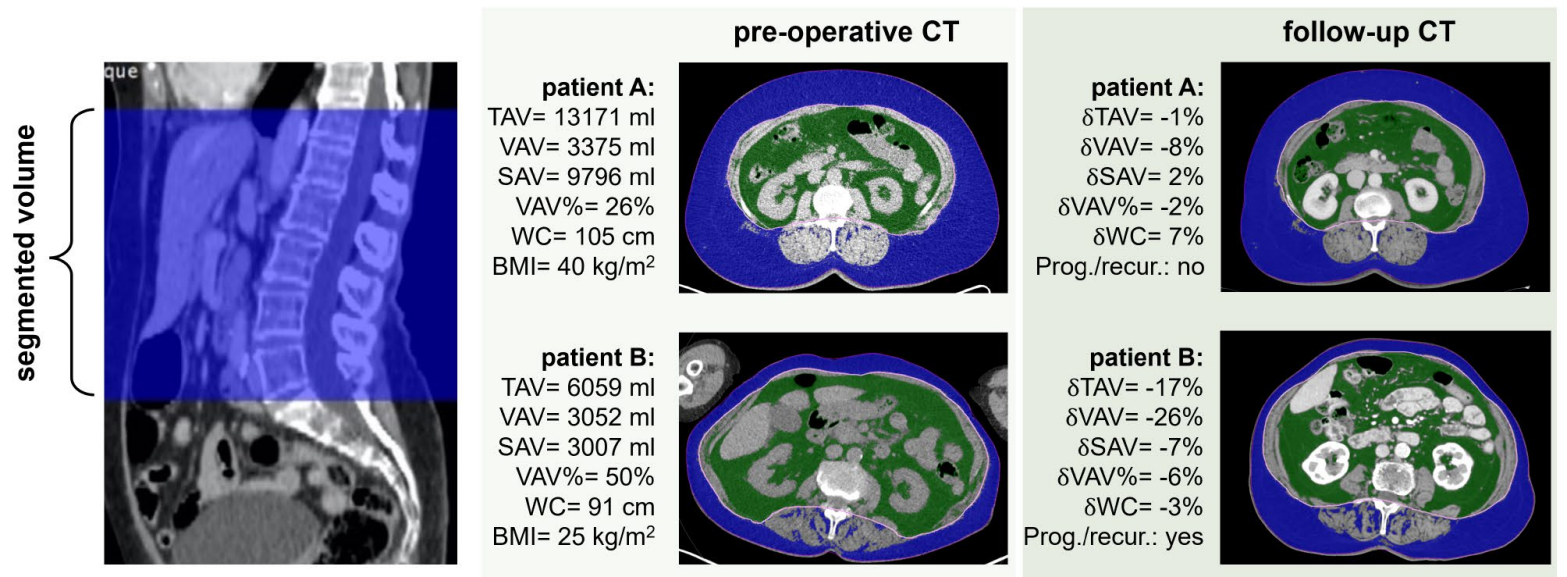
Left:* Abdominal fat compartments are derived from computed tomography (CT) scans covering the level of the upper right diaphragm to the level of vertebra L5/S1 are segmented using the software iNtuition (TeraReon Inc San Mateo, USA) *Middle*: CT obesity markers derived at primary diagnosis for patient A (63 years, FIGO IIIB, EEC grade 2, BMI 40 kg/m^2^, alive and well at follow-up) and patient B (86 years, FIGO II, NEEC, BMI 25 kg/m^2^, recurrence detected 6 months after primary treatment) *Right*: Temporal (delta, δ) changes in CT obesity markers from primary treatment to follow-up for patient A and B BMI, body mass index, EEC, endometrioid endometrial carcinoma; NEEC, non-endometrioid endometrial carcinoma; FIGO, international federation of gynecology and obstetrics; SAV, subcutaneous abdominal fat volume; TAV, total abdominal fat volume; VAV, visceral abdominal fat volume; VAV%, visceral-to-total abdominal fat percentage; WC, waist circumference at L3/L4
